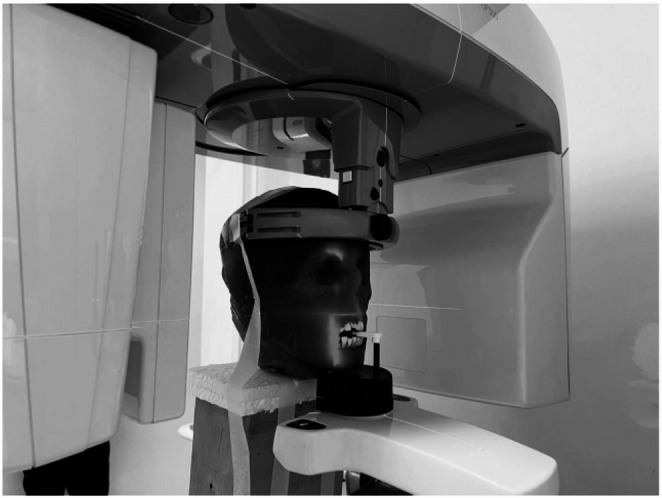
Model positioned in the panoramic radiographic unit
